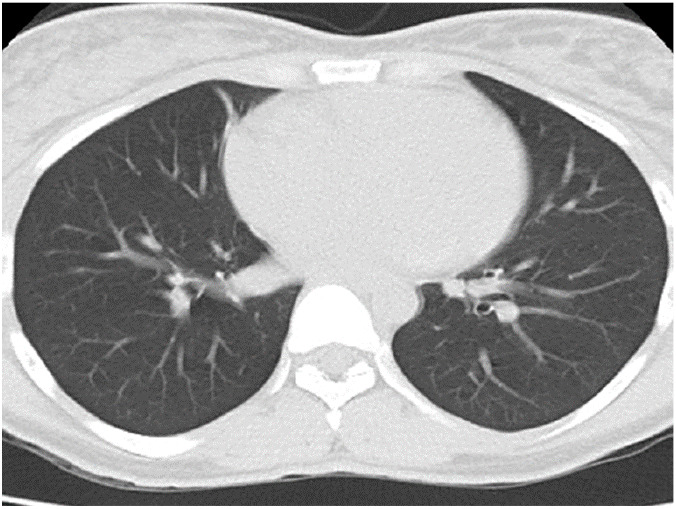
An axial non-contrast enhanced chest CT scan of a 36-year-old female with severe respiratory distress, fever, cough and anosmia of 9 days duration. Lung window image shows no parenchymal abnormality. Patient tested positive for COVID-19 but had (normal) Negative chest CT features throughout the course of the disease.
